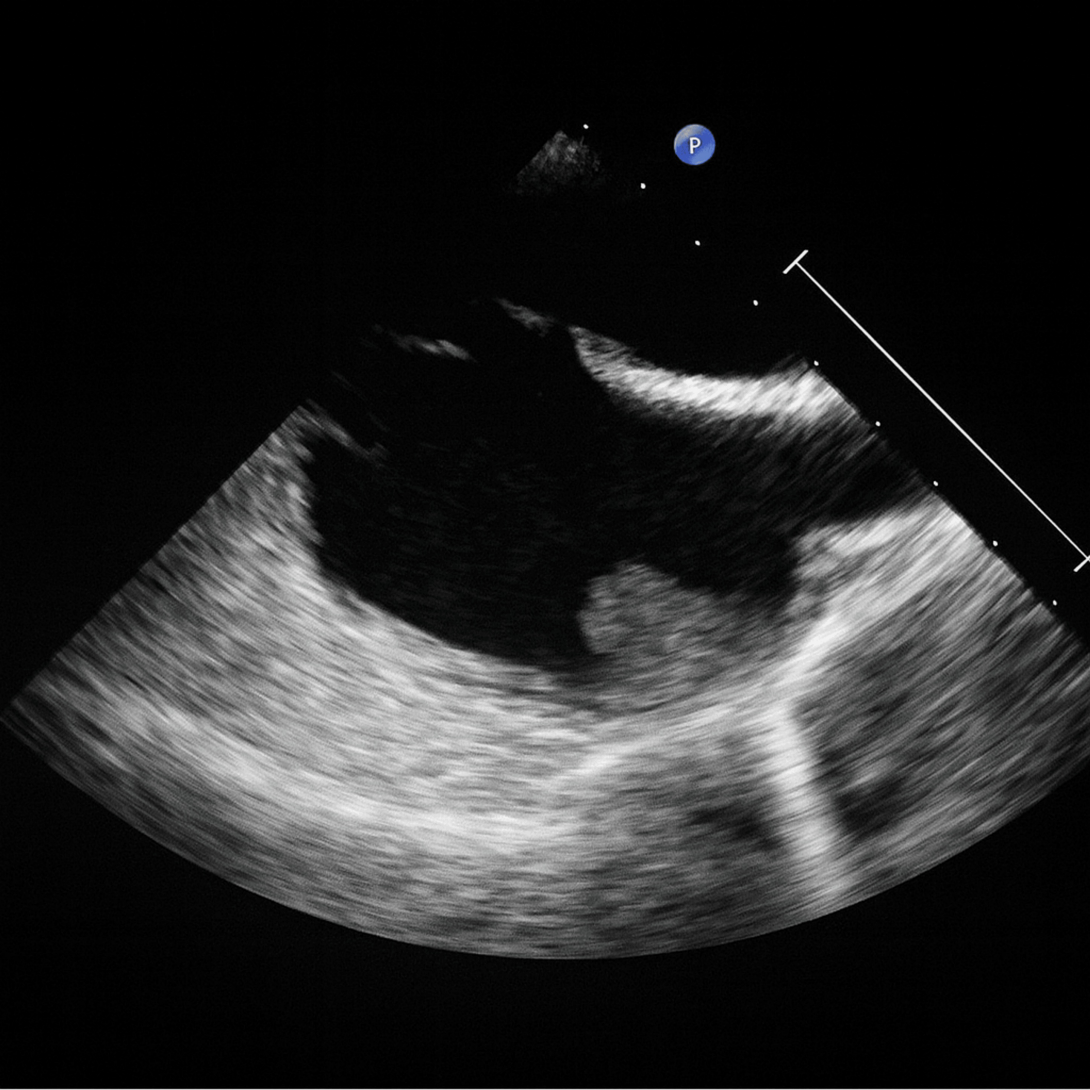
TEE bicaval view showing RAA thrombus TEE: transesophageal echocardiography; RAA: right atrial appendage
